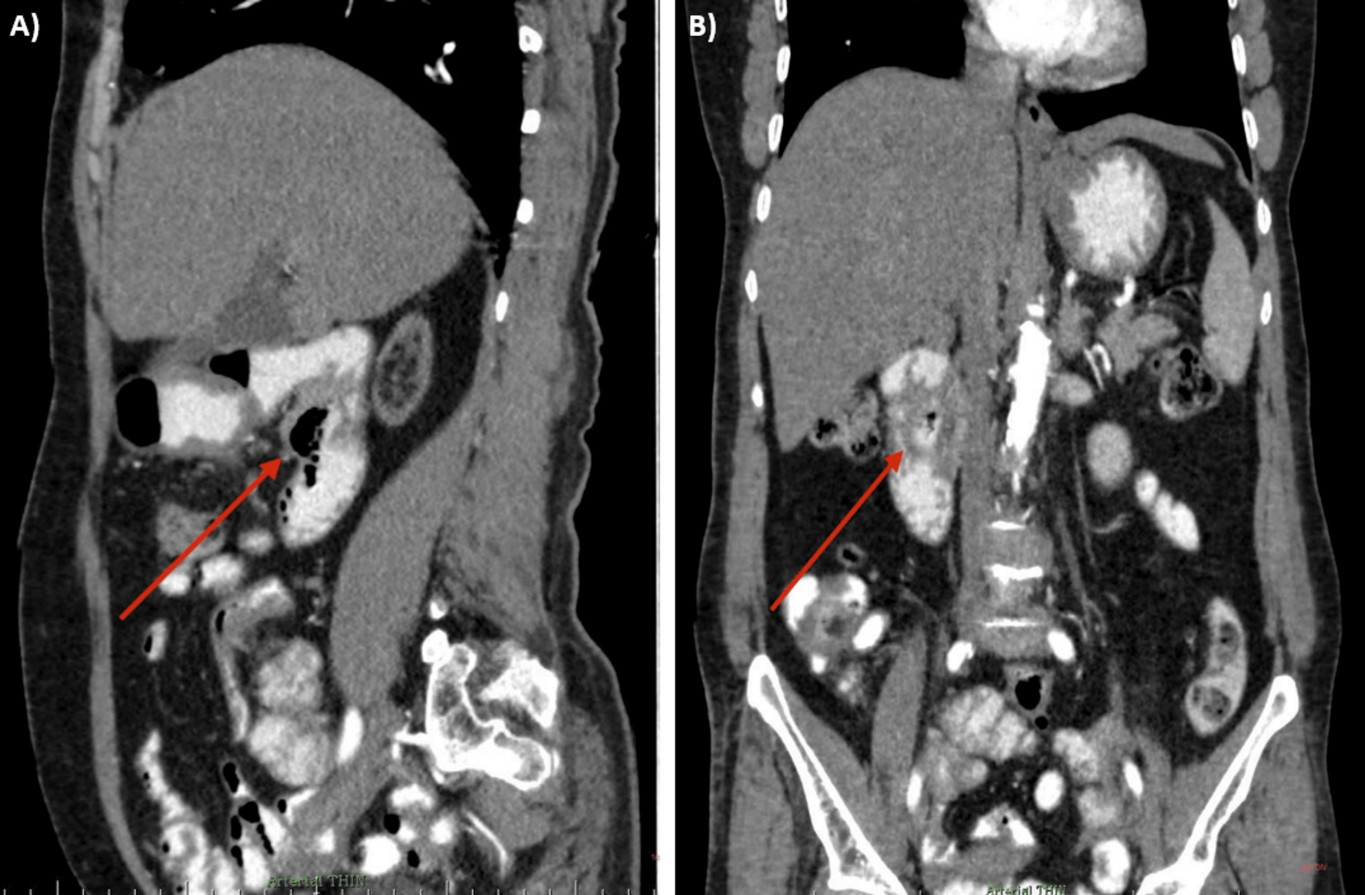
Pre-operative CECT of the abdomen and pelvis The CECT, sagittal (A) and coronal (B) views, show a large per-ampullary diverticulum measuring 4x3 cms (red arrow) causing an obstruction of the distal CBD with upstream dilatation. CECT: contrast-enhanced computed tomography; CBD: common bile duct
